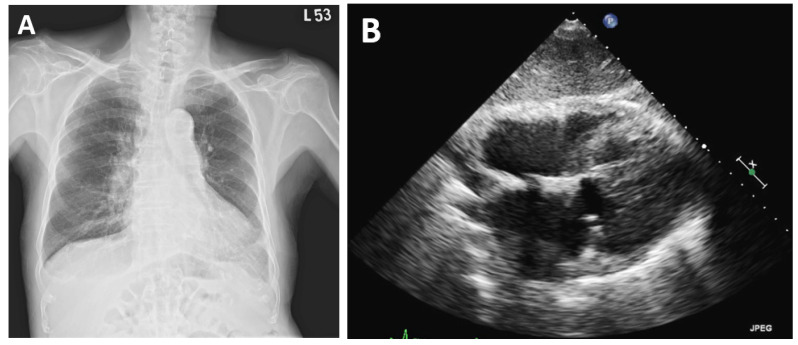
Repeated image studies during follow-up at the outpatient clinic. Chest X-ray (A) and the subcostal view of transthoracic echocardiography (B). Both show no recurrence of the effusion.
